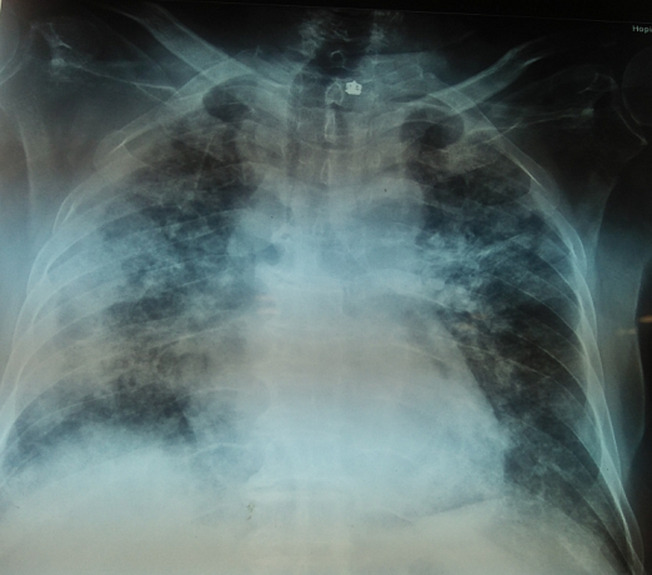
radiographie pulmonaire montrant une cardiomégalie avec un syndrome alvéolo-interstitiel bilatéral
